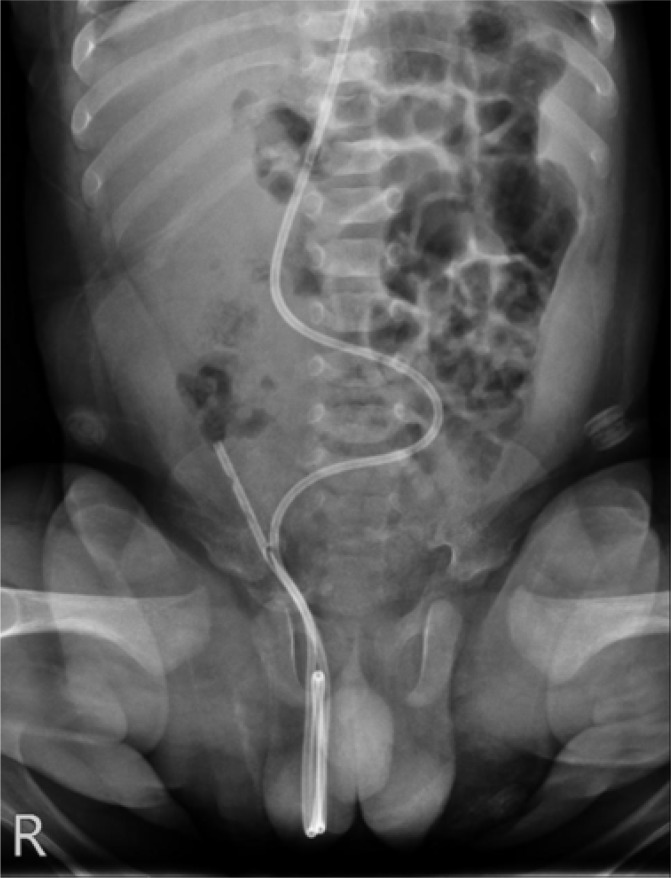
Abdominal radiography of patient in emergency department
